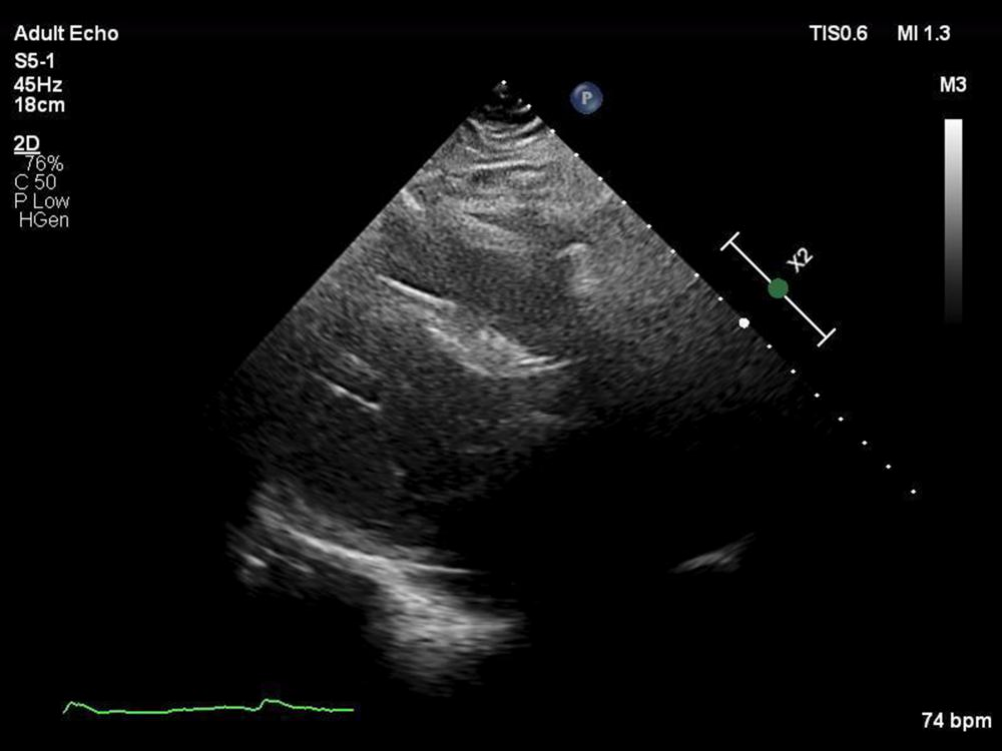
The subaortic pannus was not discernible on a parasternal long-axis view on transthoracic echocardiography, June 2022.
